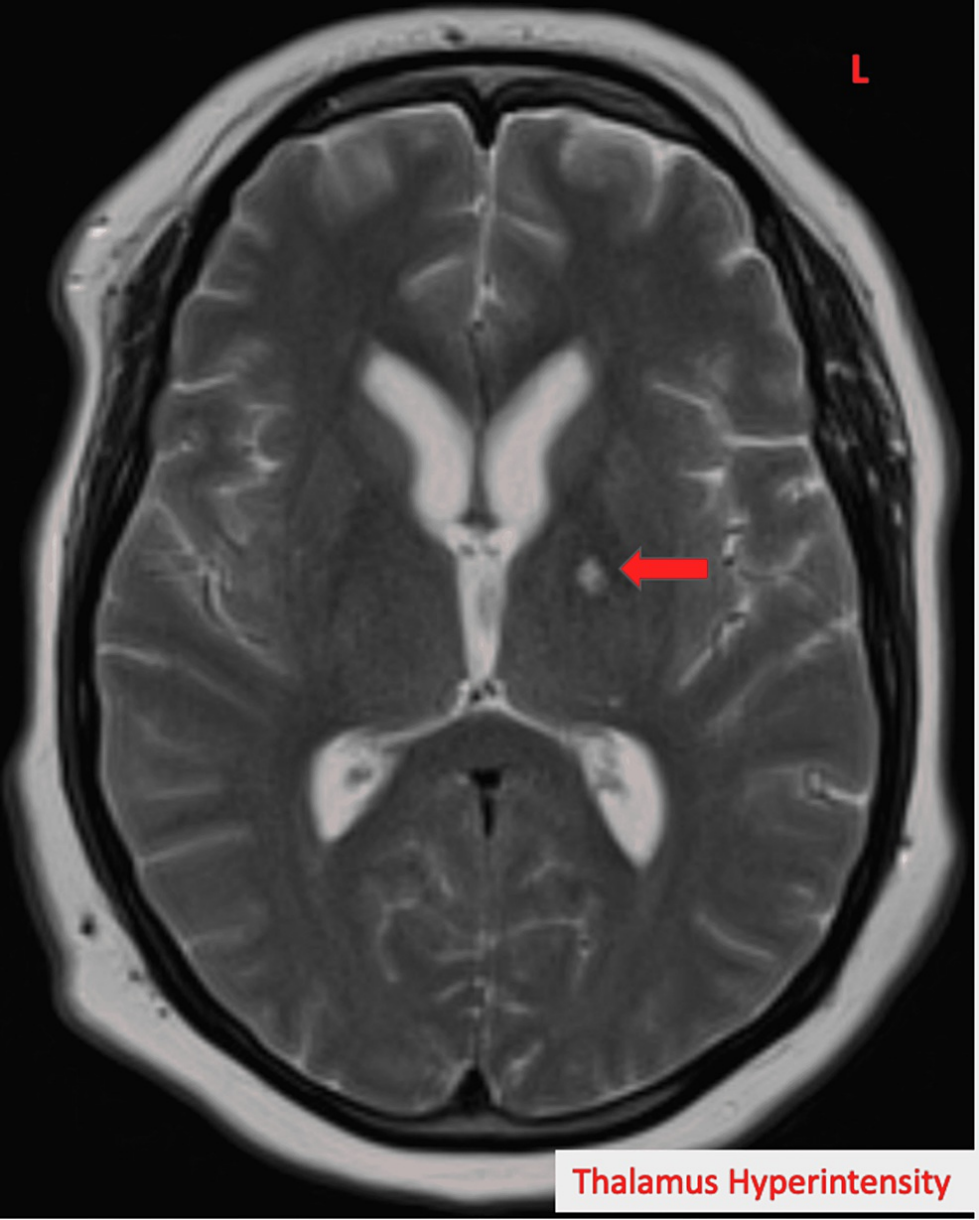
MRI brain showing hyperintensity of the left thalamus in T2 (arrow indicates the lesion).
